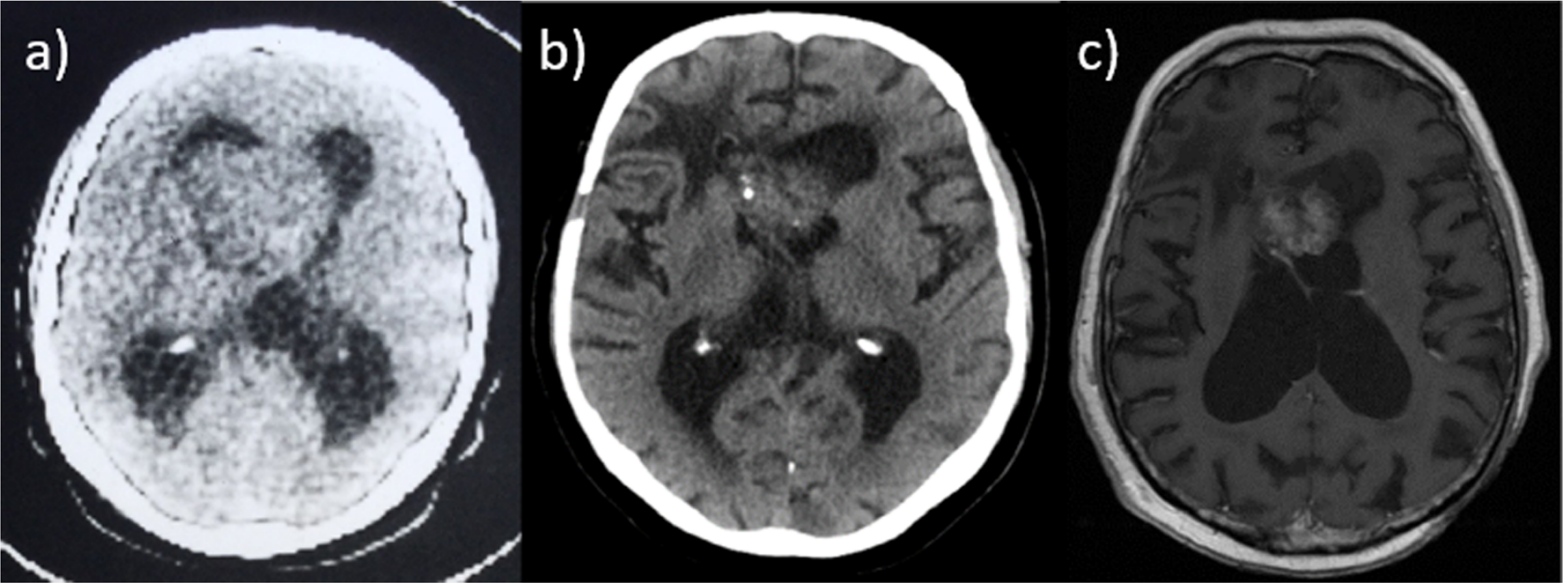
a CT scan of lateral ventricle subependymoma (taken in 1979). More recent imaging (CT (b) and MRI axial T1 post-gadolinium contrast, (c)) demonstrating stability of the subependymoma size over the long surveillance period
